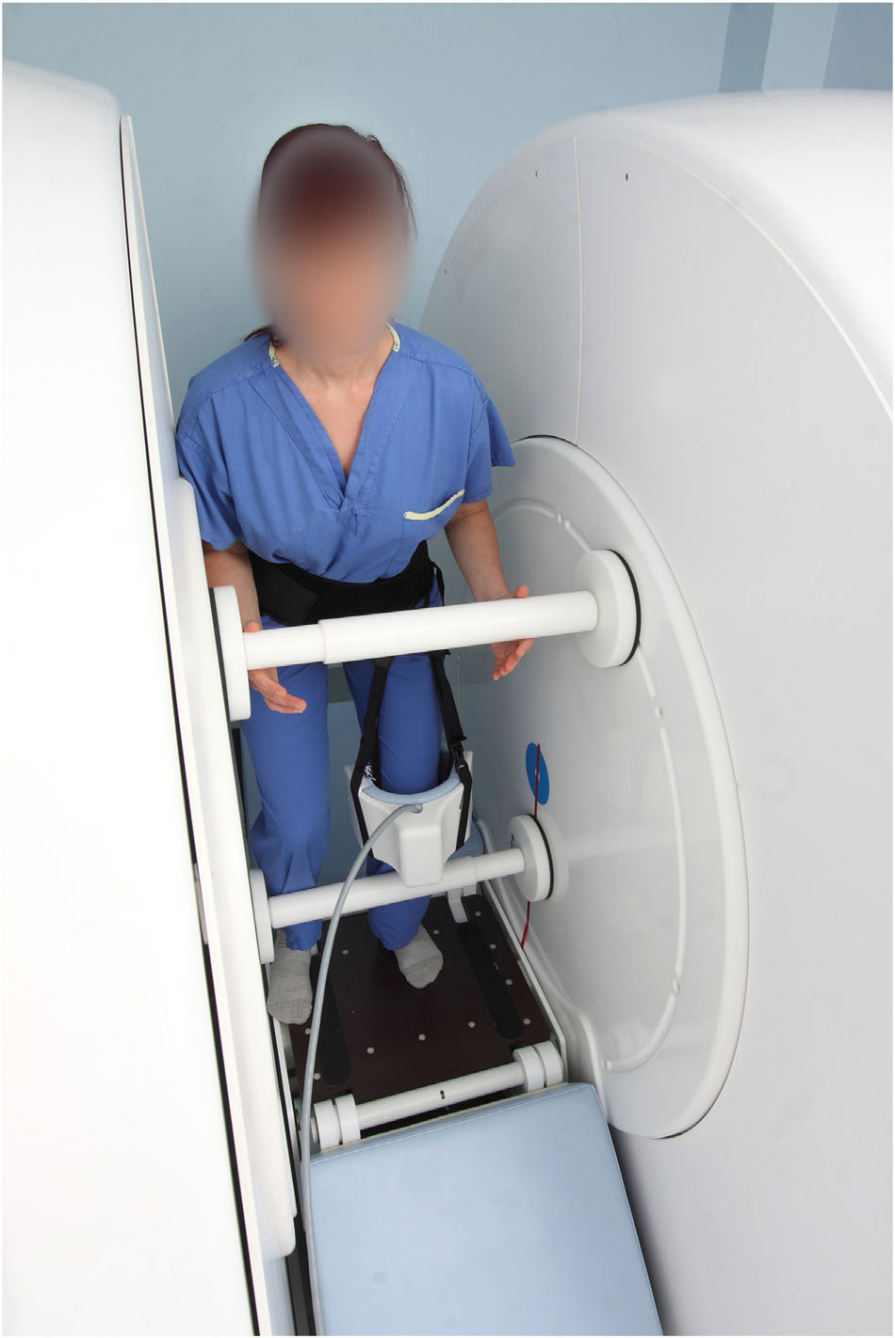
0.5T open‐bore scanner upright positioning. Participant stands on adjustable bench, a 12‐inch goniometer is used to achieve the correct knee flexion angle, then pressure bars are placed at the shins, buttocks, and at hand level for proprioceptive support, with feet positioned at 10° external rotation using a foot map (not shown). A plumb bob is used to ensure knees are not positioned anterior to the great toe. *Modified from Journal of Magnetic Resonance Imaging, Macri EM et al., “Patellofemoral and tibiofemoral alignment in a fully weight‐bearing upright MR: Implementation and repeatability” Published Online First: Doi:10.1002/jmri.25823, 2017, with permission from John Wiley and Sons*.
